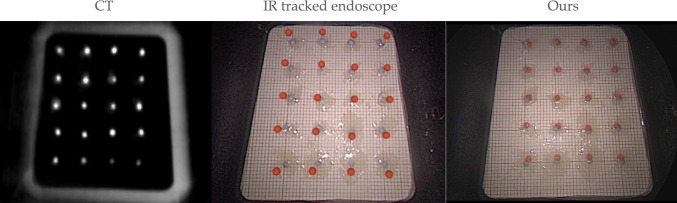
Accuracy comparison of IR-tracked AR (middle) and our system (right). Grey circles represent true target points, spaced 1 cm apart, while red dots show the AR overlay. The distance between the true target points (grey) and overlay points (red) represents the accuracy of either system. The CT scan with visible target points used for extracting red 3D locations is shown on the left
